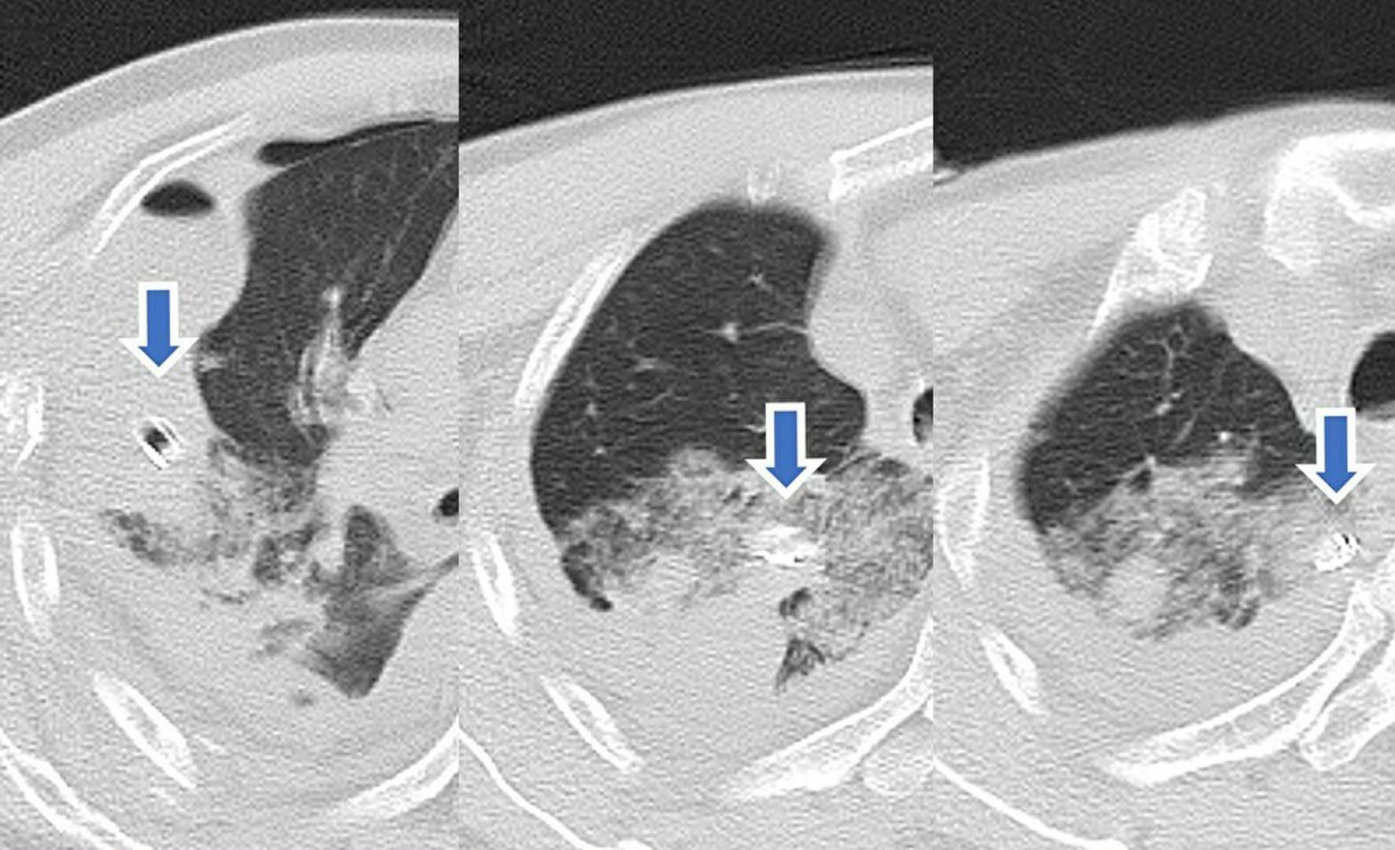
Computed tomography showing chest tube (arrows) stabbing the right upper lobe
